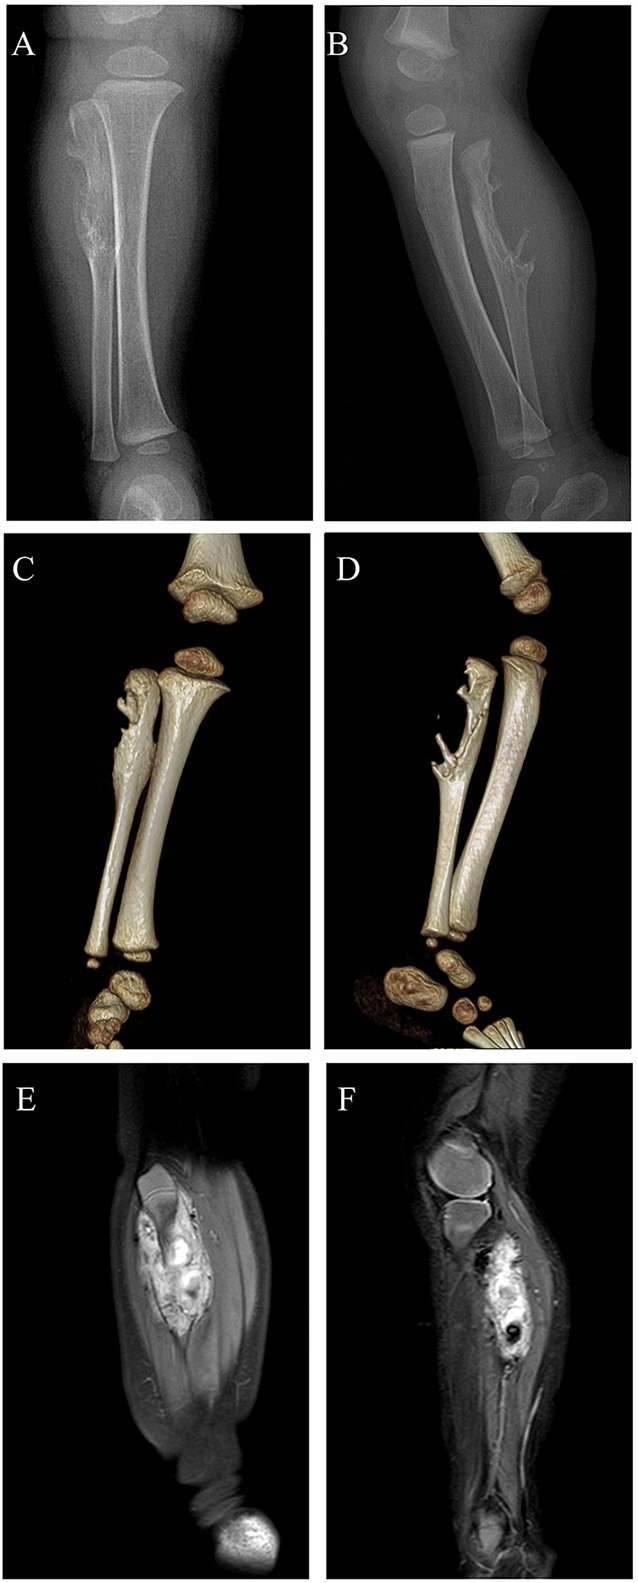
Digital radiograph preoperatively: (A,B) Radiographs of the right tibia and fibula showing an irregular bone destruction of proximal fibula; (C,D) 3d CT reconstruction demonstrating lytic bone destruction of right proximal fibula; (E,F) MRI revealing a massive vascular tumor with surrounding soft tissue hyperplasia and involvement of the proximal fibular epiphyseal plate.
